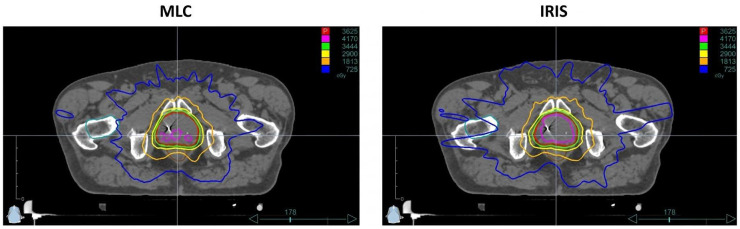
Isodose distributions for MLC and IRIS collimators on the same CT slice of a representative patient. Dose levels are shown at 100% (red), 115% (magenta), 95% (green), 80% (yellow), 50% (orange), and 20% (blue) of the prescribed dose. IRIS shows a broader high-dose spread, while MLC results in a more confined low-dose region.
